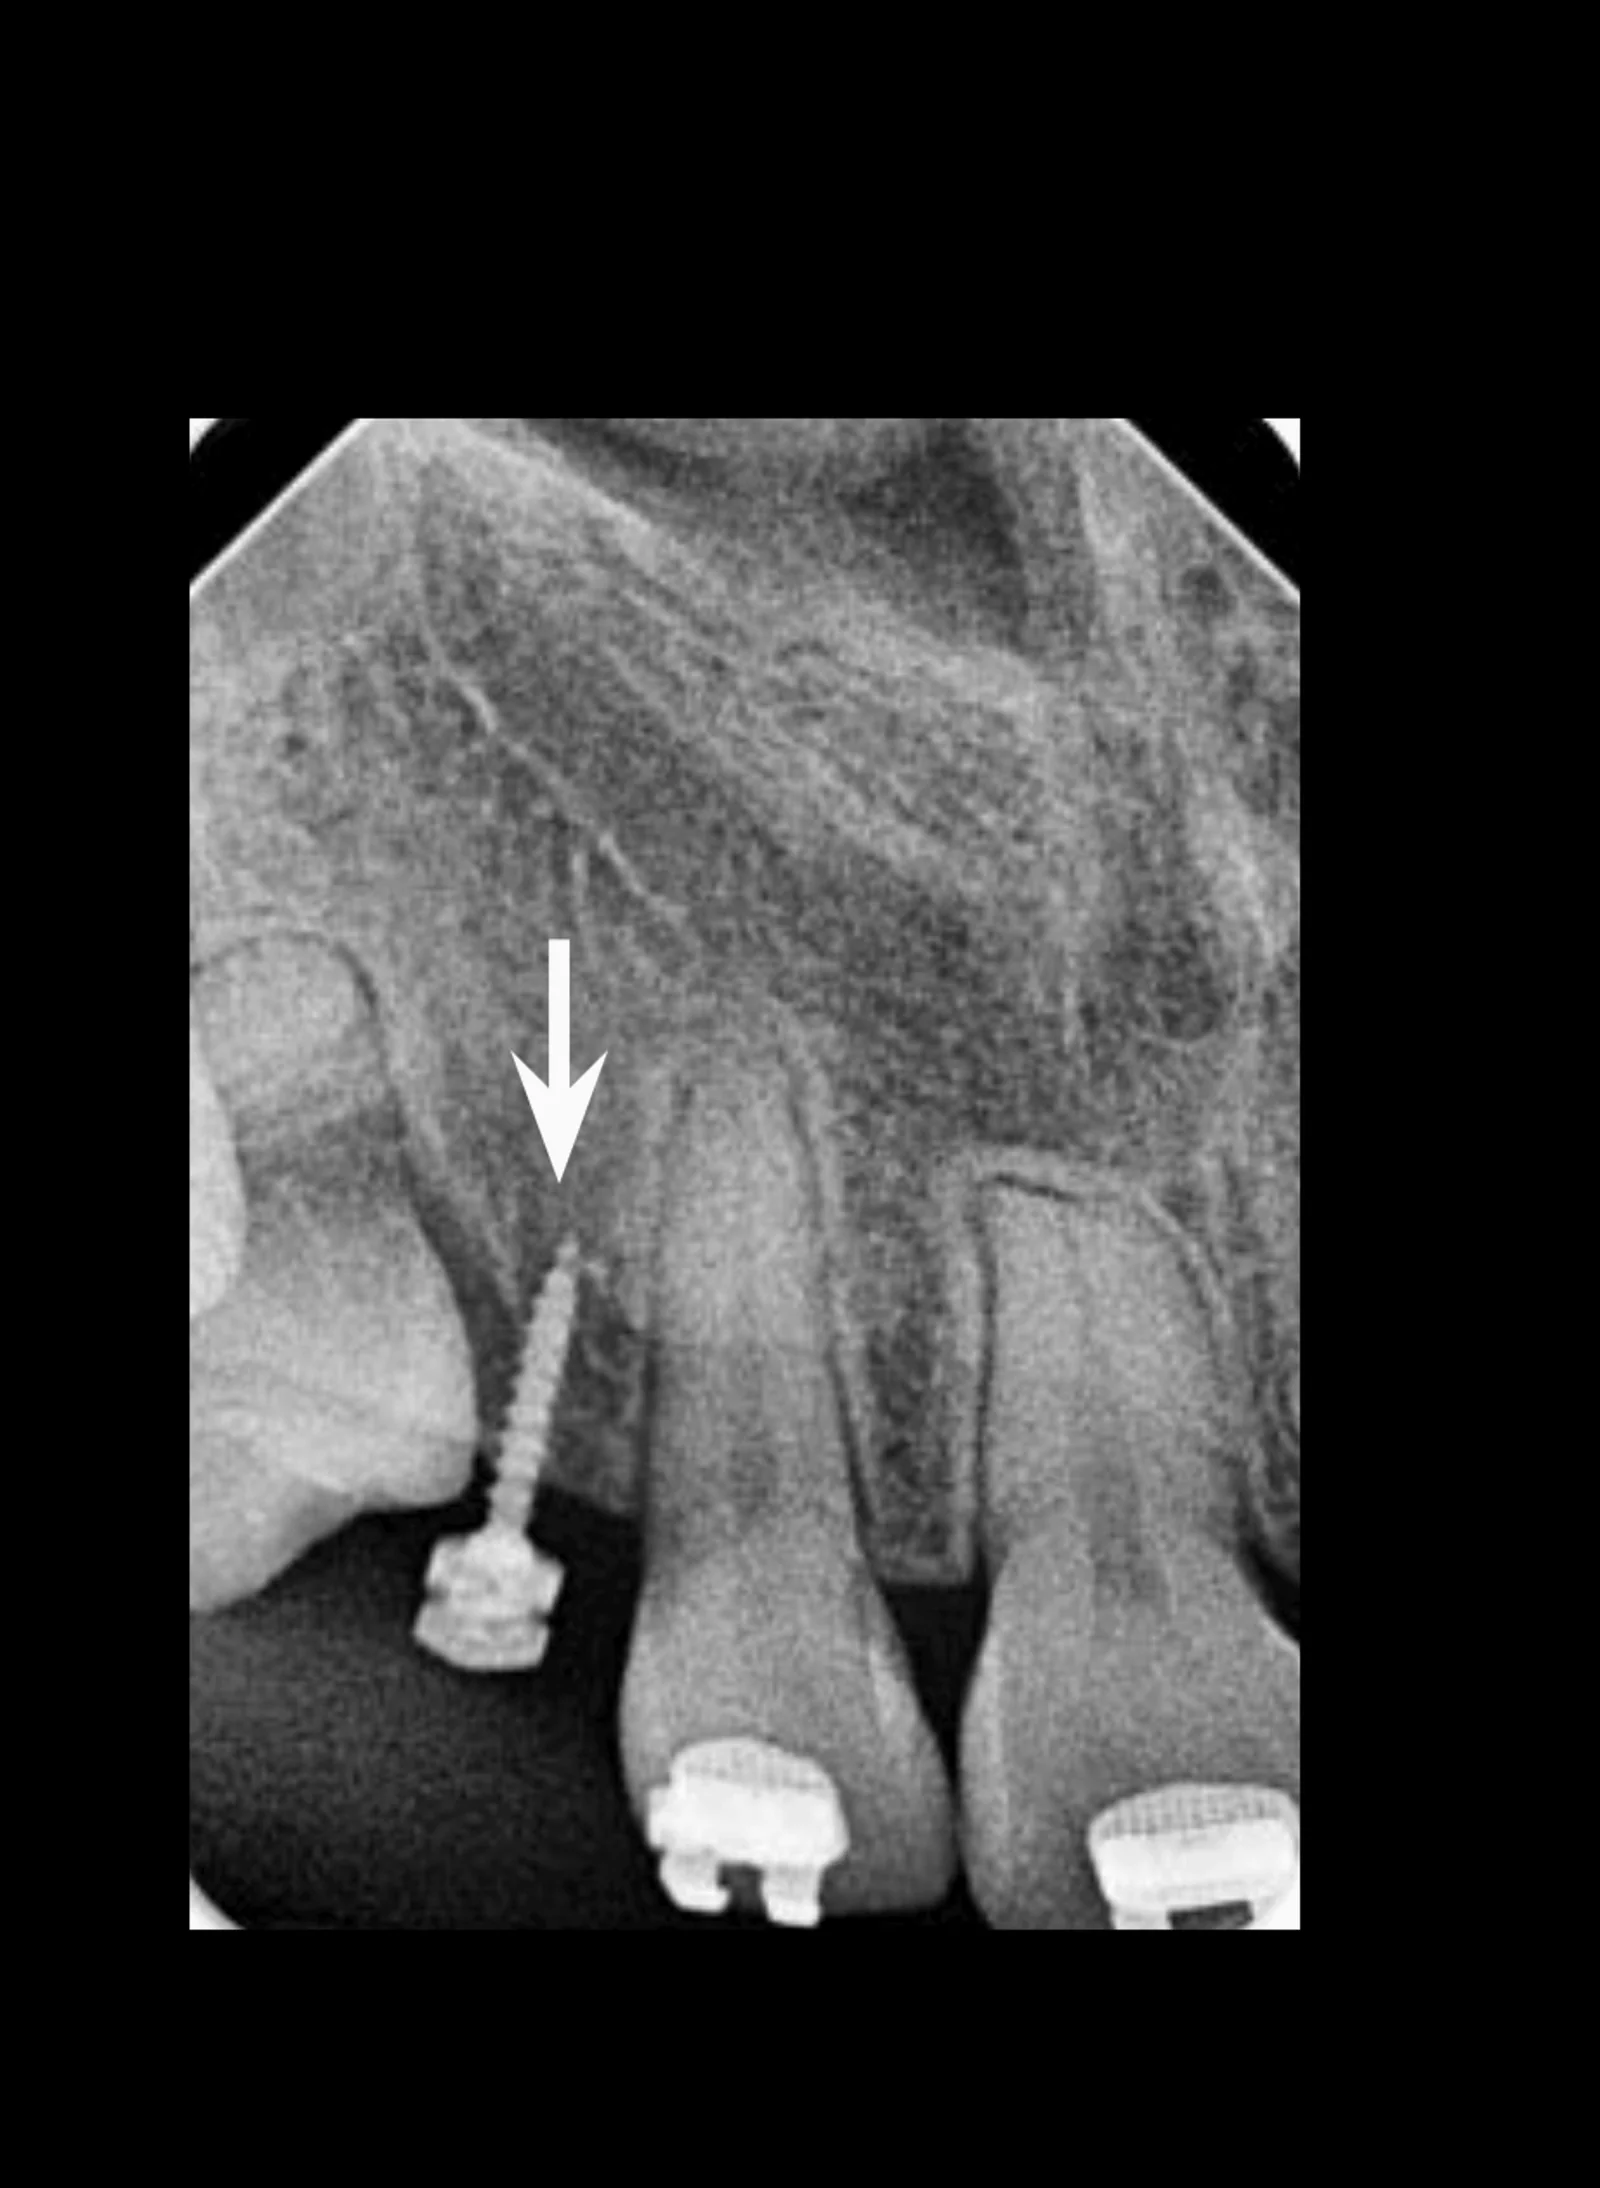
Intraoperative periapical radiograph The radiograph confirmed the position and trajectory of the microscrew relative to the adjacent root structures. The arrow indicates the microscrew placed in a parallel orientation.
